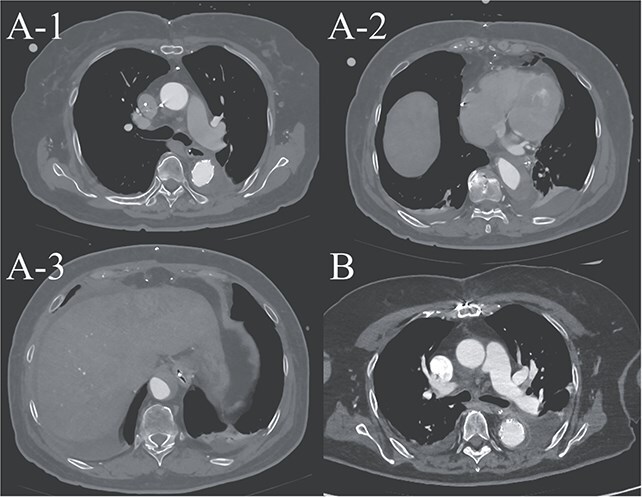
(A-1–3) contrast-enhanced CT image acquired on postoperative Day 2, showing advanced false lumen thrombosis. (B) Contrast-enhanced CT image acquired on postoperative Day 21, demonstrating further remodeling of the false lumen. This image is at approximately the same level as A-1, and the false lumen's thickness is clearly reduced.
